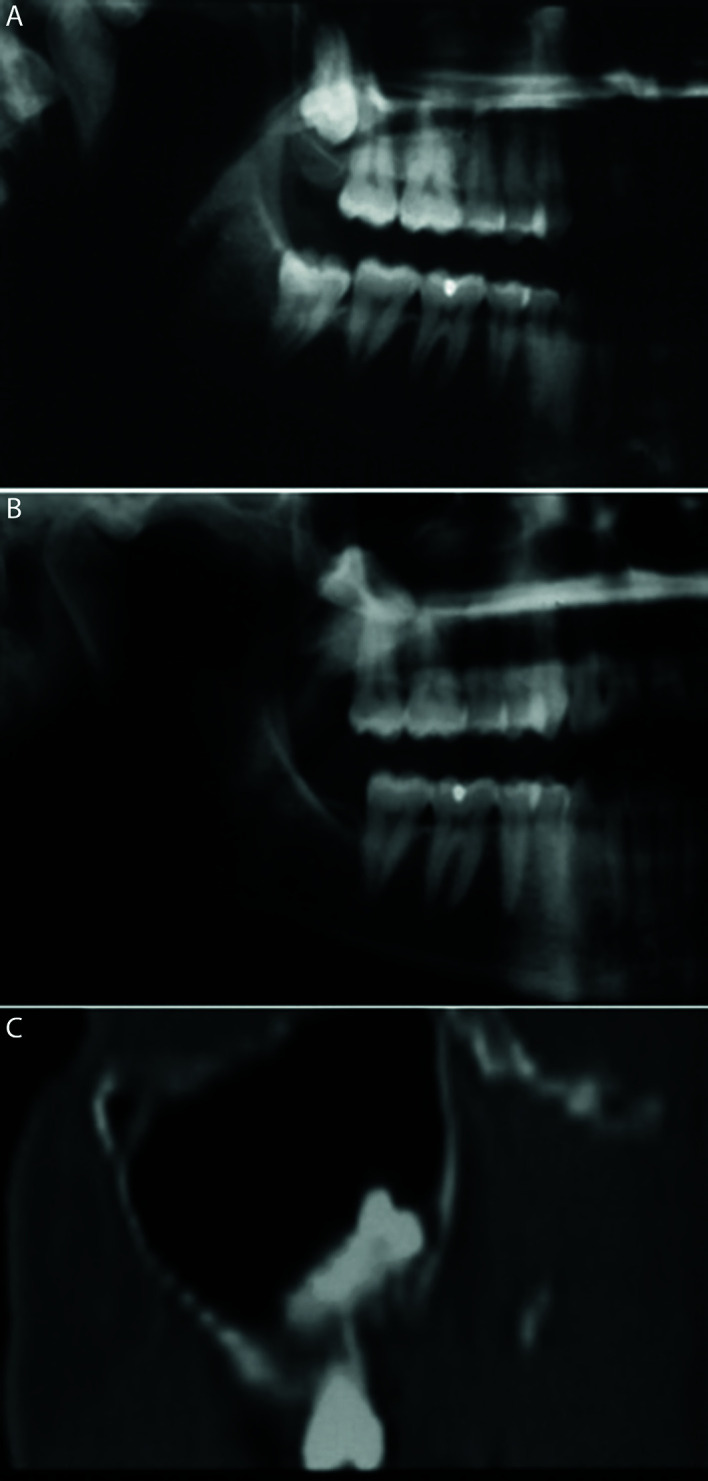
Panoramic radiograph view prior to extraction (A) and after the first attempt of removal (B). Computed tomographic view (coronal section) showing 1.8 displaced into the right maxillary sinus (C).
